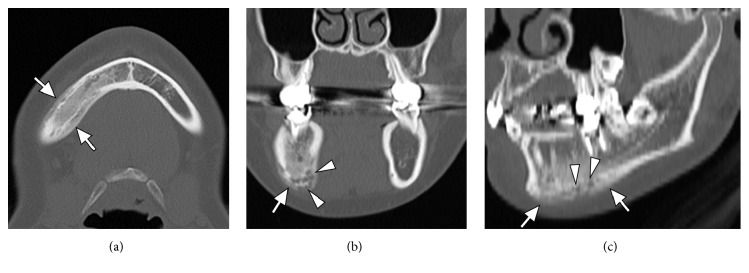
CT scan of the mandible. Axial (a) and coronal (b) and oblique sagittal reconstruction (c) plain CT images show sclerotic change and periosteal reaction in the right body of the mandible (arrows). Spotted osteolysis is also seen mainly in the cortical bone (arrowheads).
